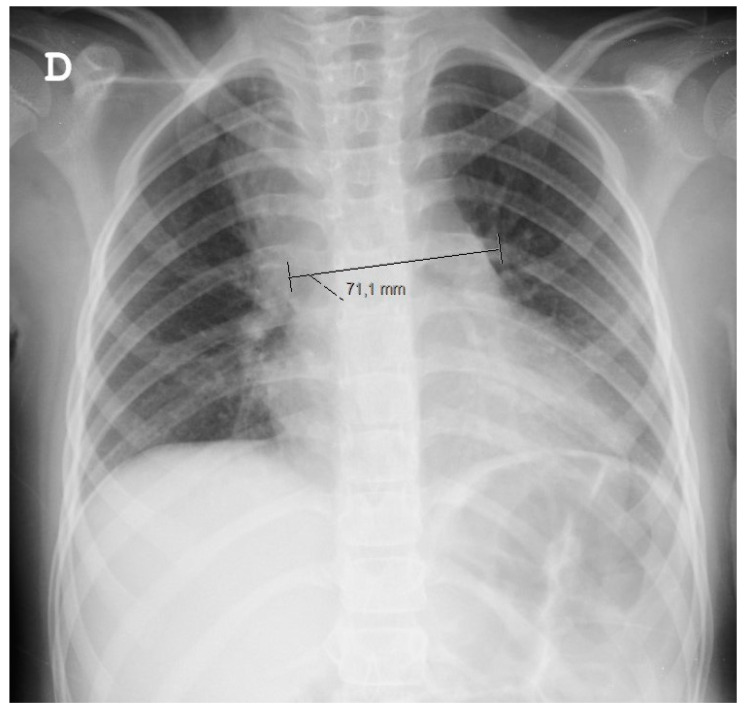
Chest radiograph. D stands for “direita”—right in Portuguese.
